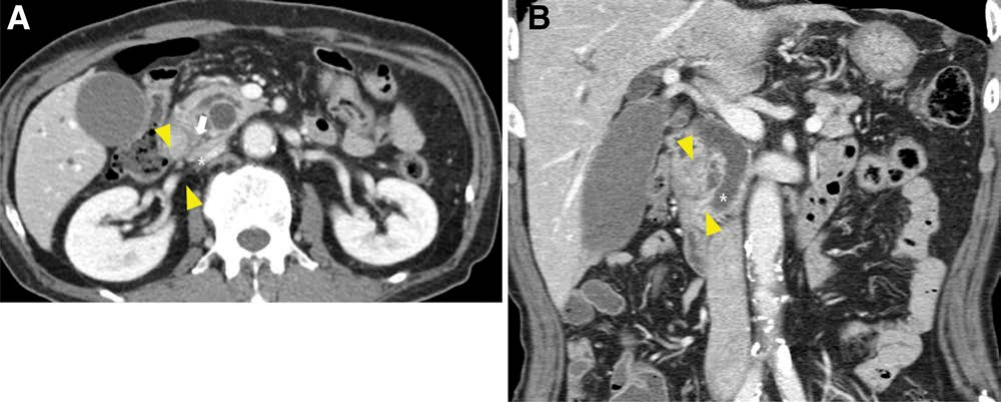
An ill-defined low-density lesion (arrowheads), with a small cystic portion in the pancreatic head, is suggestive pancreatic cancer. This is accompanied by a double-duct sign dilatation of the main pancreatic duct (arrow) and the common bile duct (asterisk), as observed on axial (A) and coronal (B) CT images. CT = computed tomography.
